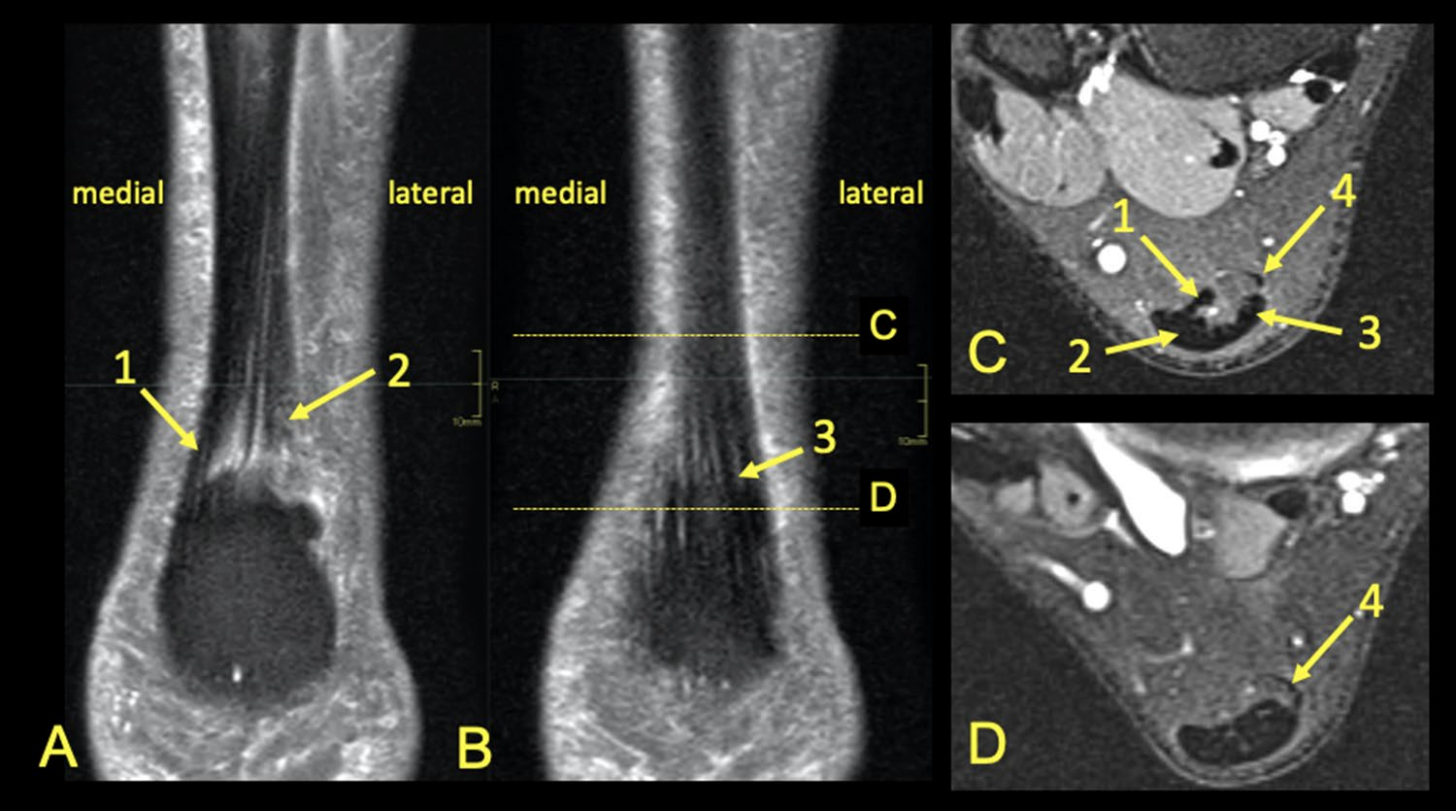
MRI right Achilles tendon, PD FS. The words medial and lateral are given for better orientation. (A,B) coronal picture of the right Achilles tendon. (C,D) transverse section at level shown on (B). 1—fibers from the soleus muscle, 2—fibers from the lateral head of the gastrocnemius muscle, 3—fibers from the medial head of the gastrocnemius muscle, 4—the plantaris tendon.
